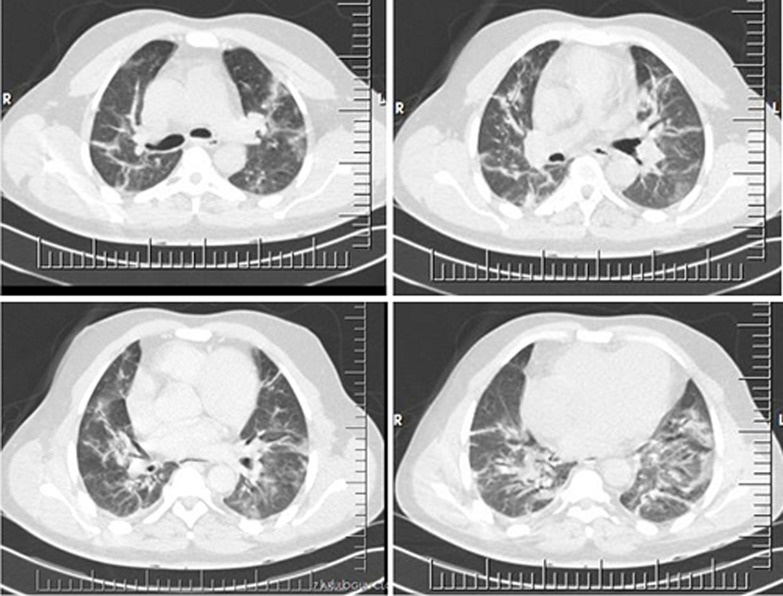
axial CT scan (lung window) showing diffuse peripherally and basally-distributed ground-glass densities with consolidations in both lungs
